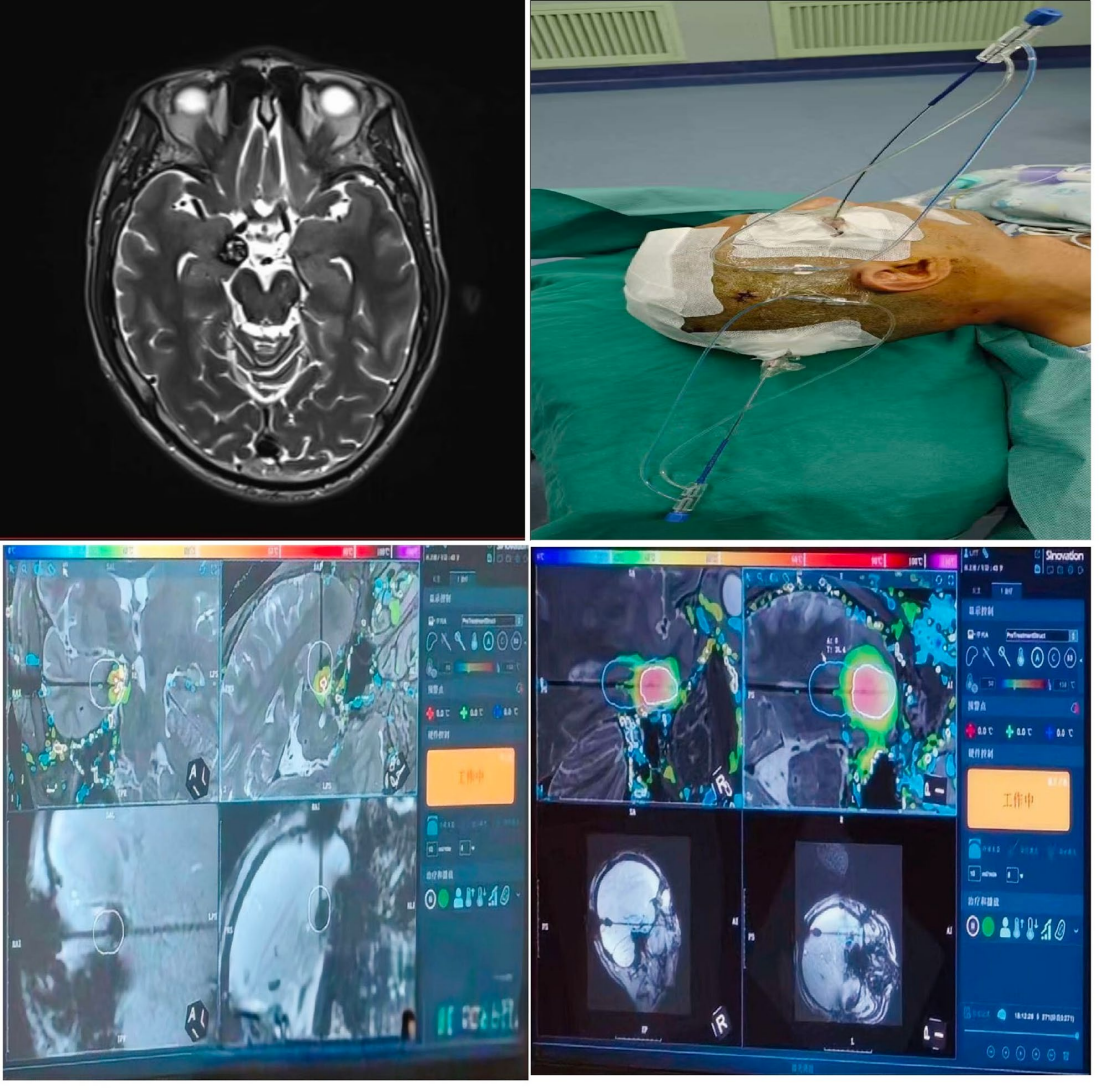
The MR image of intracranial lesion, the patient with two intracranial fiber-optic probes, and the images of MR guided treatment
